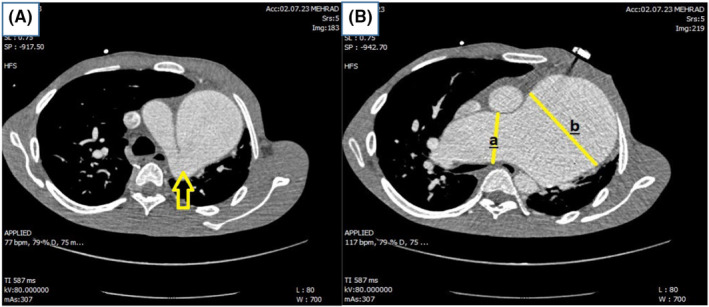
(A) PDA that was a connection between main PA and descending aorta (arrow) in this patient, (B) aneurysmal dilation of main PA (line b: 9 cm) and RPA (line a: 6.2 cm) in CT angiography.
